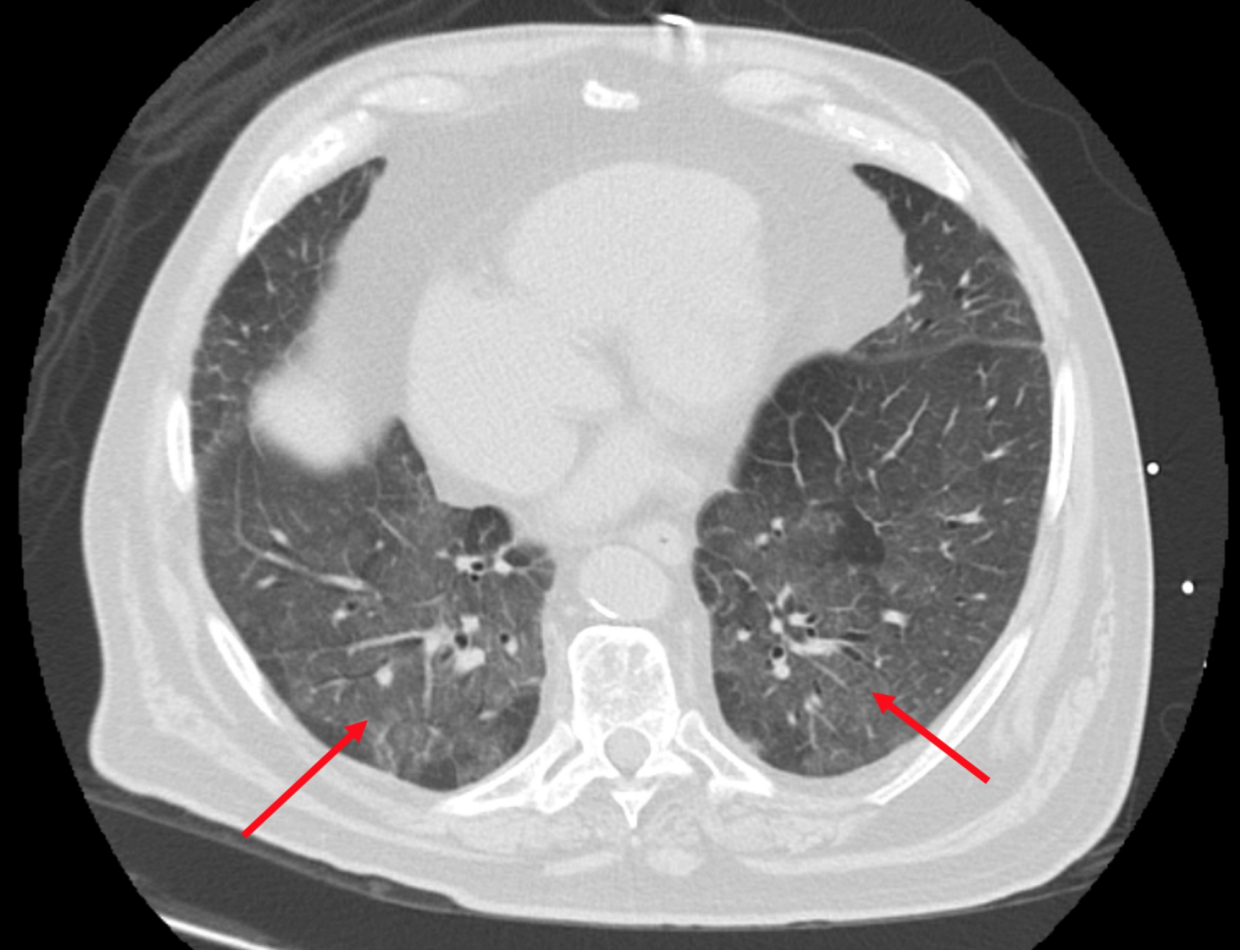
Computed tomography chest, axial image showing bilateral ground glass opacities (red arrows) with peripheral and basilar predominance typical of COVID-19.
